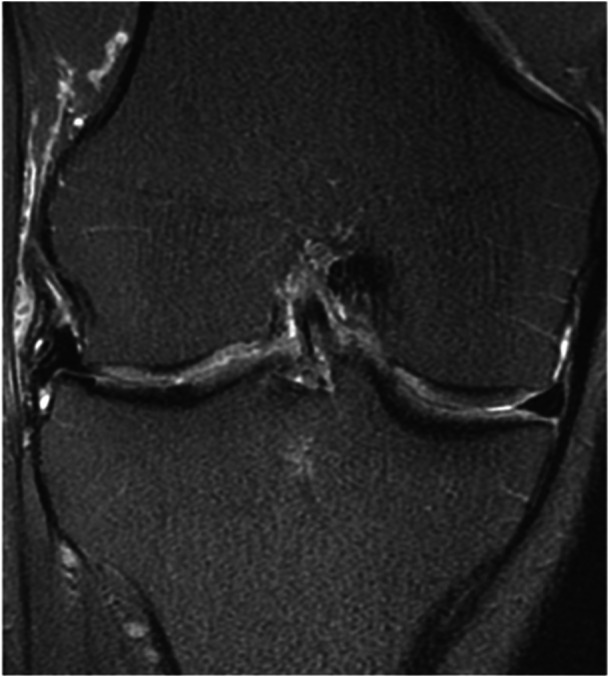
MRI coronal view of a right knee at 5 years follow‐up post lateral meniscal transplantation. An almost complete meniscal body extrusion can be observed. Despite this radiological finding the patient is completely asymptomatic. MRI, magnetic resonance imaging.
